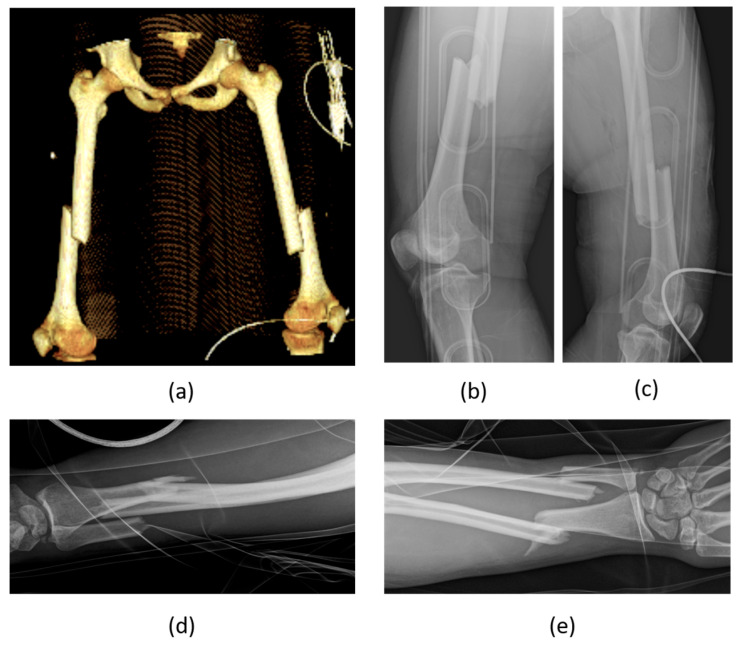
Young male patient, 20 years old, admitted for a polytrauma in an MVA (motor vehicle accident)—3D-CT reconstruction of the lower extremities with diaphyseal bilateral femur fractures (a), X-rays of the right femur (b), left femur (c), and left forearm fracture, lateral (d), and ap view (e). Young male patients are more commonly involved in MVAs compared to their female counterparts and trauma is one of the most common causes of death in this age class and gender.
